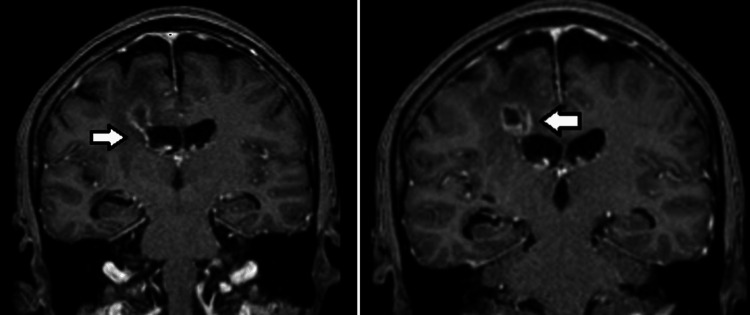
Brain T1-weighted MRI showing a peripheral contrast uptake e signs of diffusion restriction, suggesting purulent content associated to perilesional edema (arrow on left and right images)
